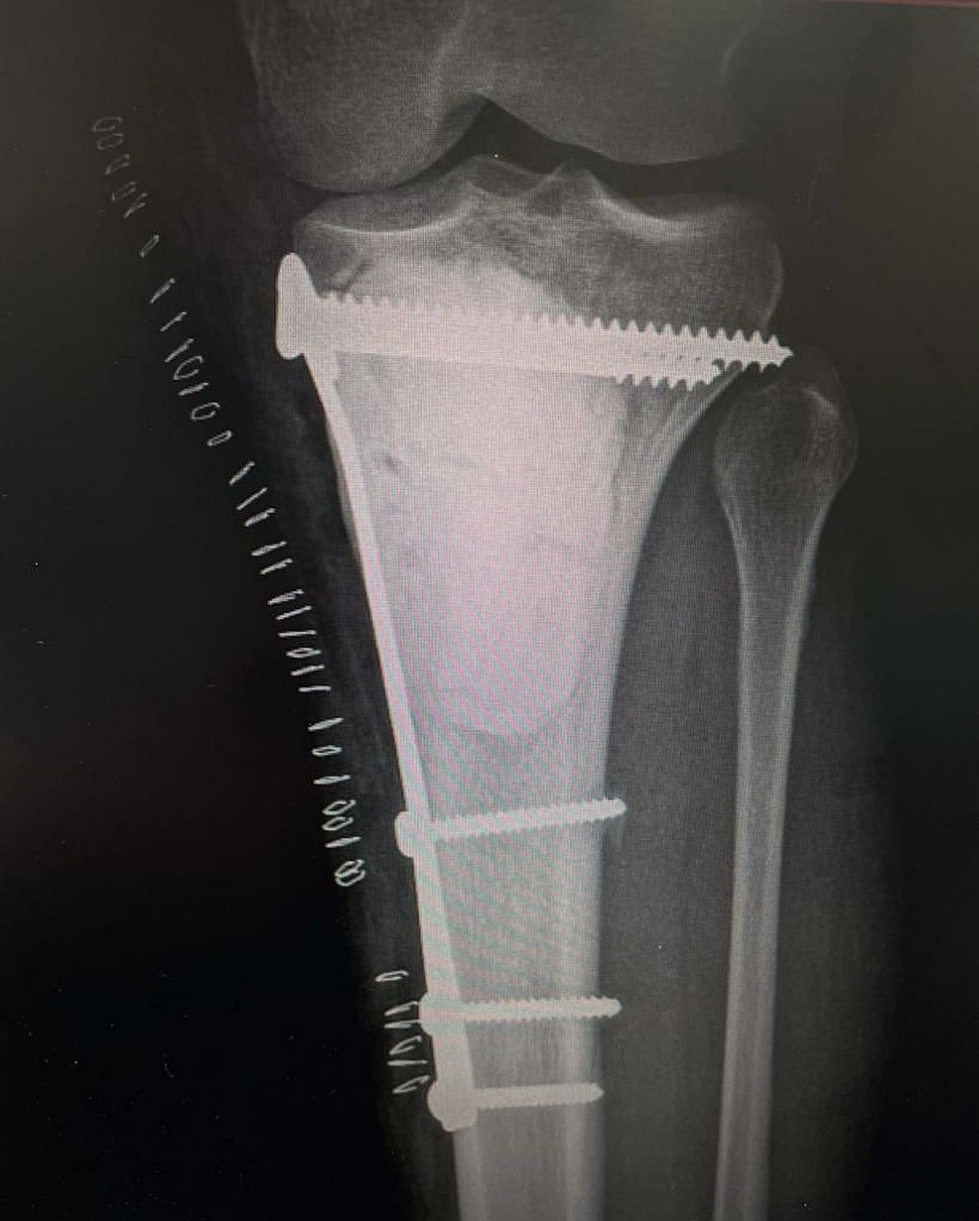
Post-operative x-rays showing the bone which was filled with cement after supporting the subchondral surface by an allograft layer of bony chips and fixed with a supportive medial side plate.
